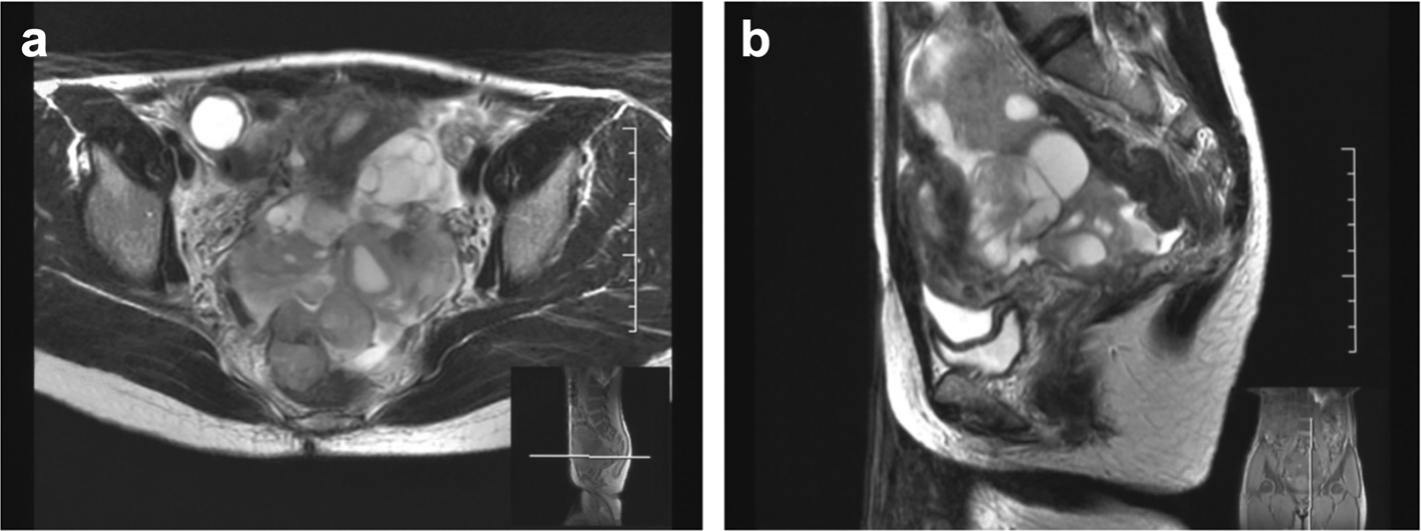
Pelvic magnetic resonance imaging scans showing advanced low-grade endometrial stromal sarcoma 10cm in diameter behind the uterus with signal intensities from low to high. a T2-weighted axial slice. b T2-weighted sagittal slice
